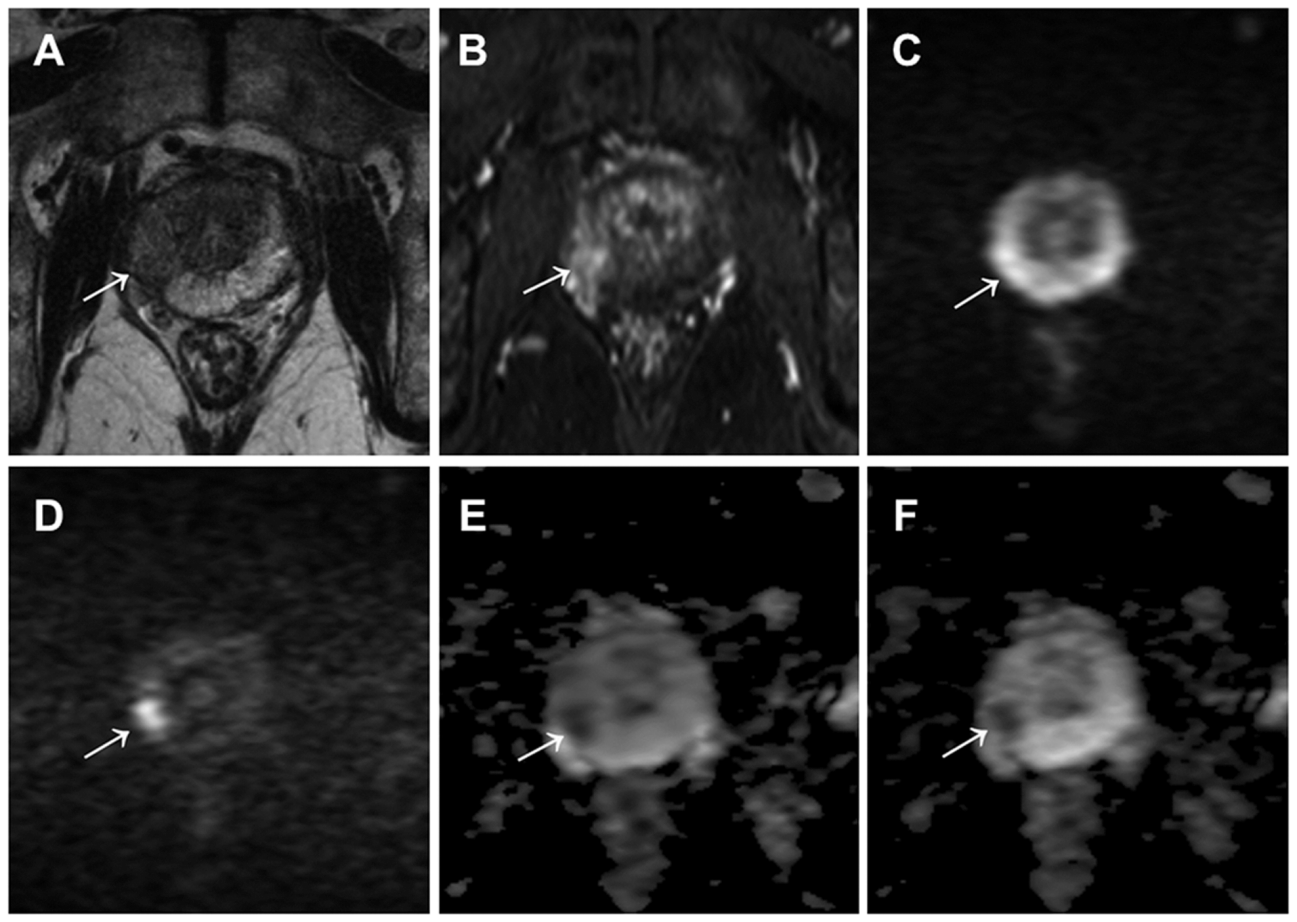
A 77-year-old male with prostate cancer (PSA level of 7.45 ng/mL, Gleason score of 4+3) in the middle right region in the peripheral zone. Cancer lesion is shown as a homogeneous hypointense lesion with mass effect on T2-weighted imaging (arrow) (A) and focal early enhancement on dynamic contrast-enhanced MR imaging (arrow) (B). The lesion with a focal hyperintensity is depicted clearly in the DW image with b values of 0 and 2000 s/mm^2^ (arrow) (D), as compared with the DW image with b values of 0 and 1000 s/mm^2^ (arrow) (C). However, the lesion conspicuity with a focal hypointensity in the ADC map with b values of 0 and 2000 s/mm^2^ (arrow) (F) was equivalent to that of ADC map with b values of 0 and 1000 s/mm^2^ (arrow) (E).
